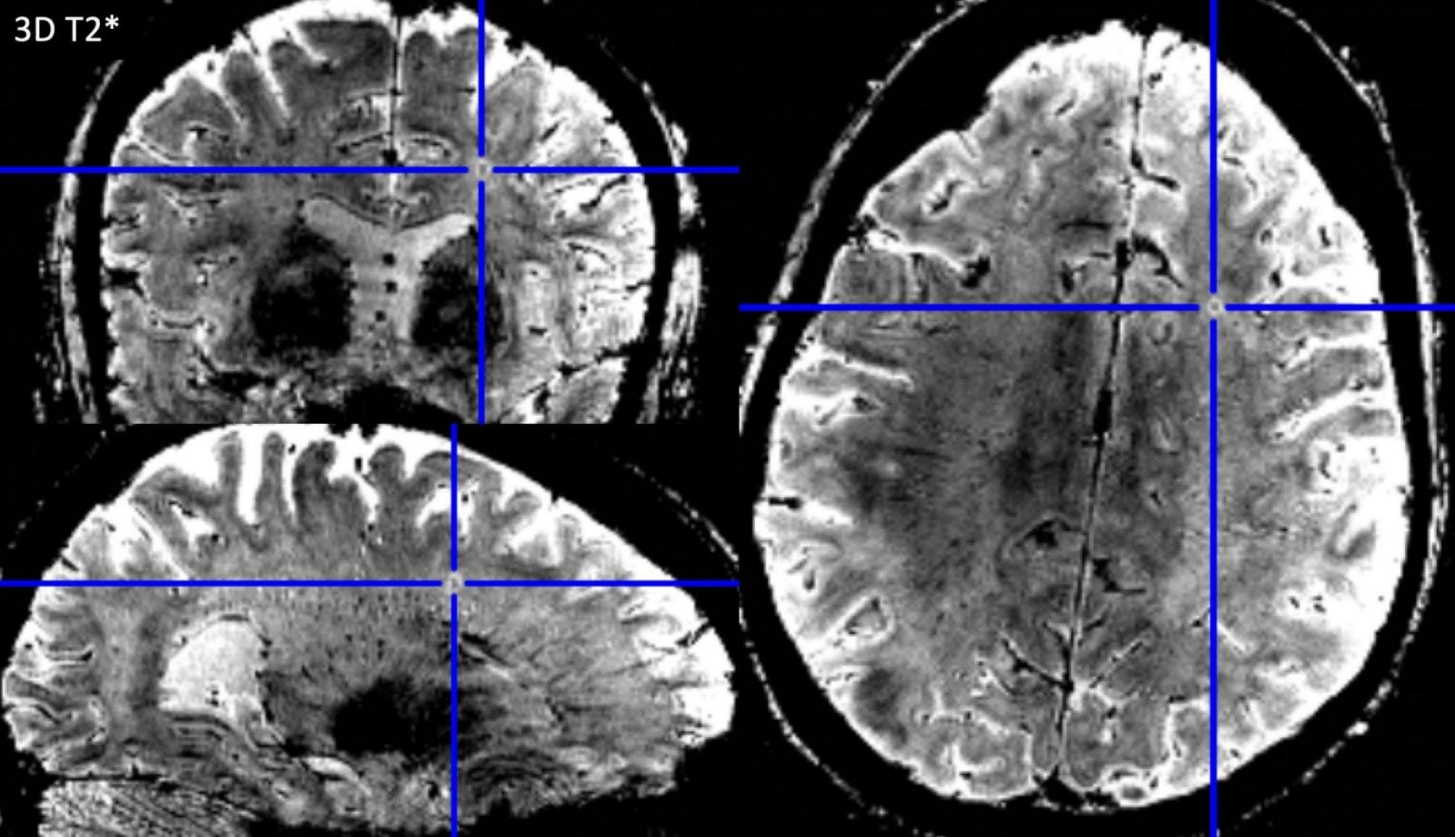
“Iron dot” lesion in the left frontal lobe on 3D T2* weighted coronal (top left), sagittal (lower left), and axial (right) 7 Tesla magnetic resonance imaging sequences. Please note the strong but punctate and sharply delineated intra-lesional signal loss on T2*w. This type of intra-lesional T2*w signal loss differs from that of more recent multiple sclerosis (MS) lesions where central T2*w hypointensities appear larger and more diffuse. They are also distinct from (enlarged) venules present in MS lesions as “iron dot” lesions do not show a tubular appearance following the course of a vessel
